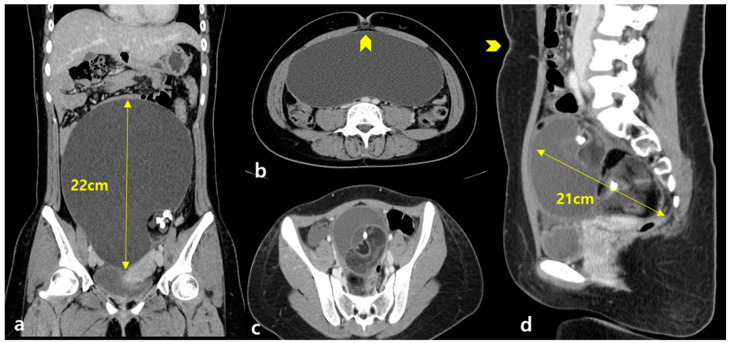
(a) A 17-year-old woman with a huge abdominal mass (Case 1). A coronal view on computed tomography (CT) revealed calcification within a 22 × 20 cm ovarian mass with low signal intensity on T2 weighted imaging (T2WI), suggesting a mature cystic teratoma. (b) An axial view, umbilicus (arrowhead) (c) of an 18-year-old woman with bilateral huge abdominal masses (Case 2). An axial view revealed calcification within a 17 × 15 cm ovarian multi-cystic mass and another 8 × 7 cm mass with low signal intensity on T2WI, suggesting a mature cystic teratoma. (d) A sagittal view, umbilicus (arrowhead).
